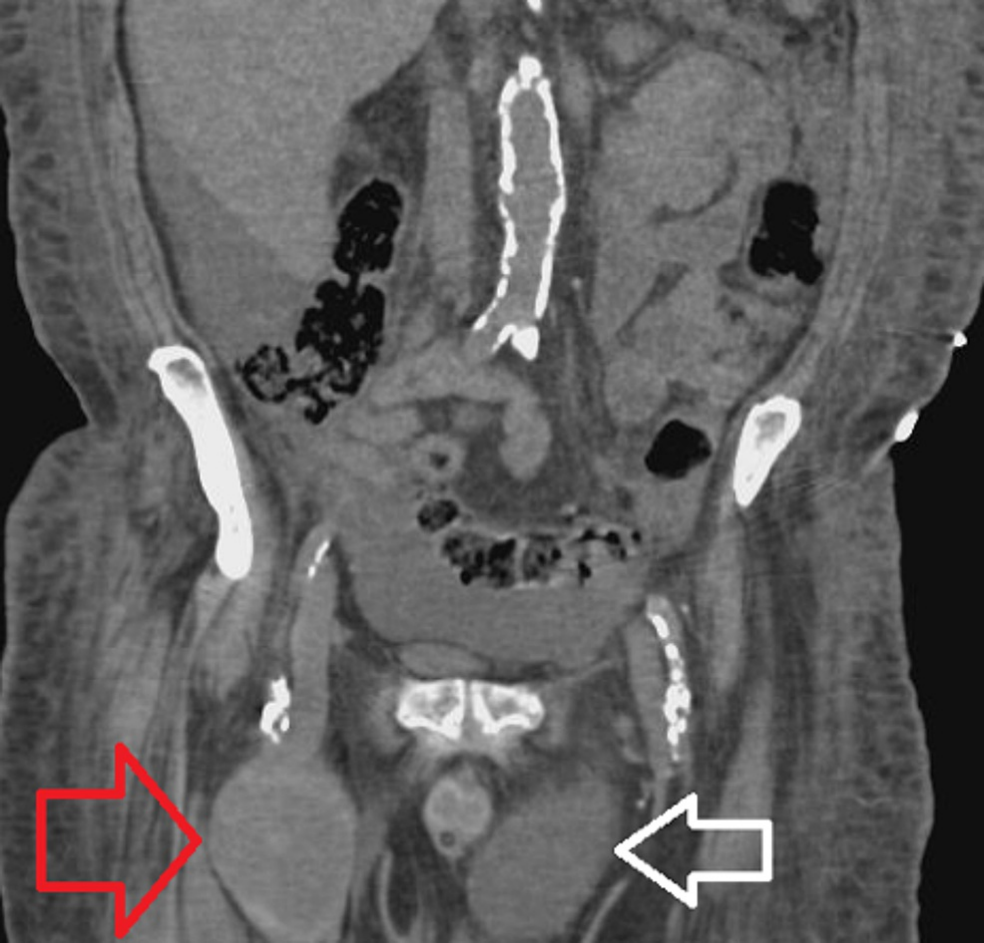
Coronal CT scan without IV contrast. Red arrow showing the large superficial femoral pseudoaneurysm. White arrow showing the large left inguinal hernia.
